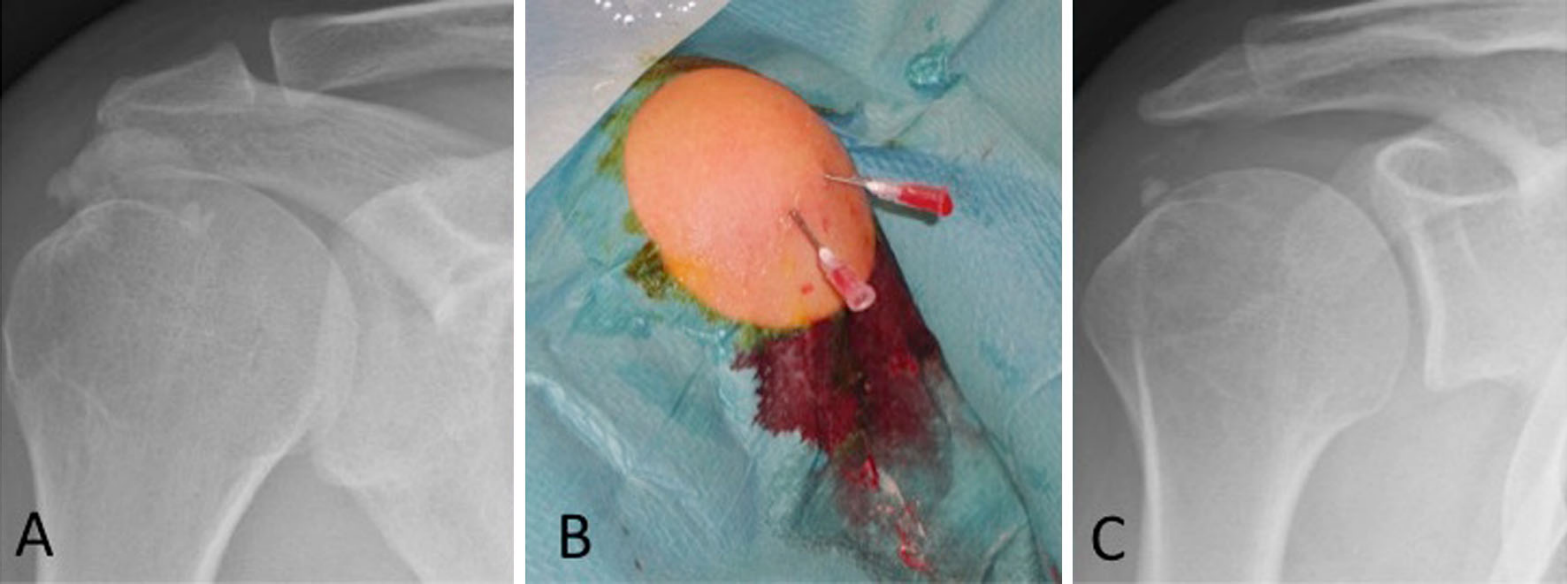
a AP view radiograph shows a big calcium deposit (>1 cm) of the supraspinatus (SS) tendon in a case with acute phase, b image of the same case who underwent ultrasound guided needling and bursal lavage of the subacromial space with leakage of copious amounts of semisolid calcium deposits, c X-ray performed after 2 months from bursal lavage showed almost complete resorption of the calcium deposit
